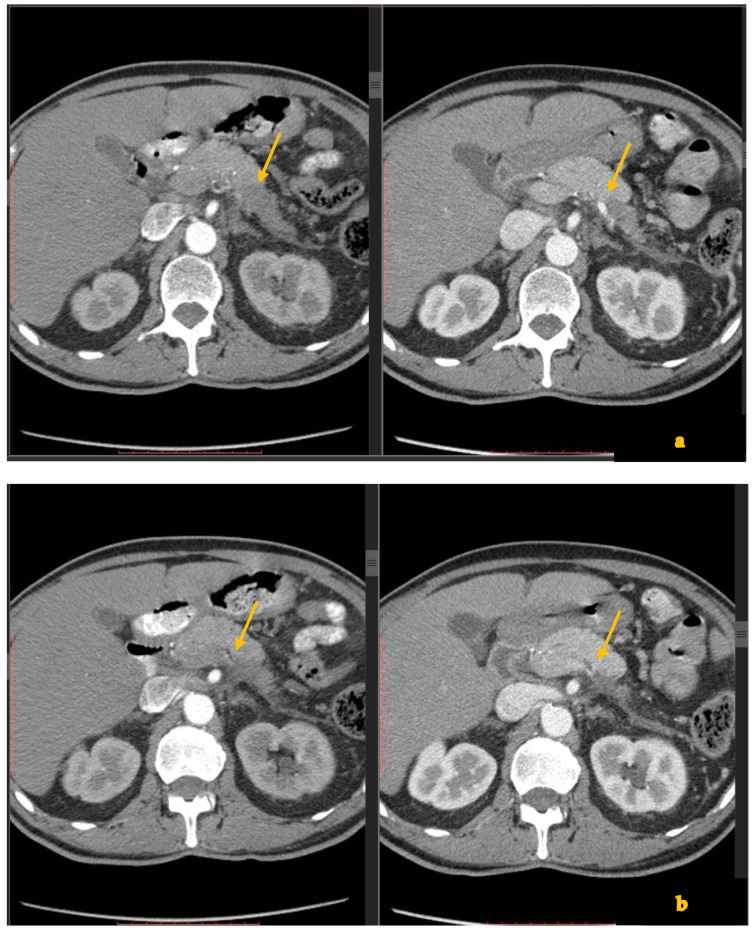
(a,b) Contrast-enhanced CT shows a large mass (arrows) in the pancreatic body and tail (a). After chemotherapy (b), there is improvement, but there is still visible tissue (arrows) close to the superior mesenteric artery, which was proven on surgery to represent fibrosis.
